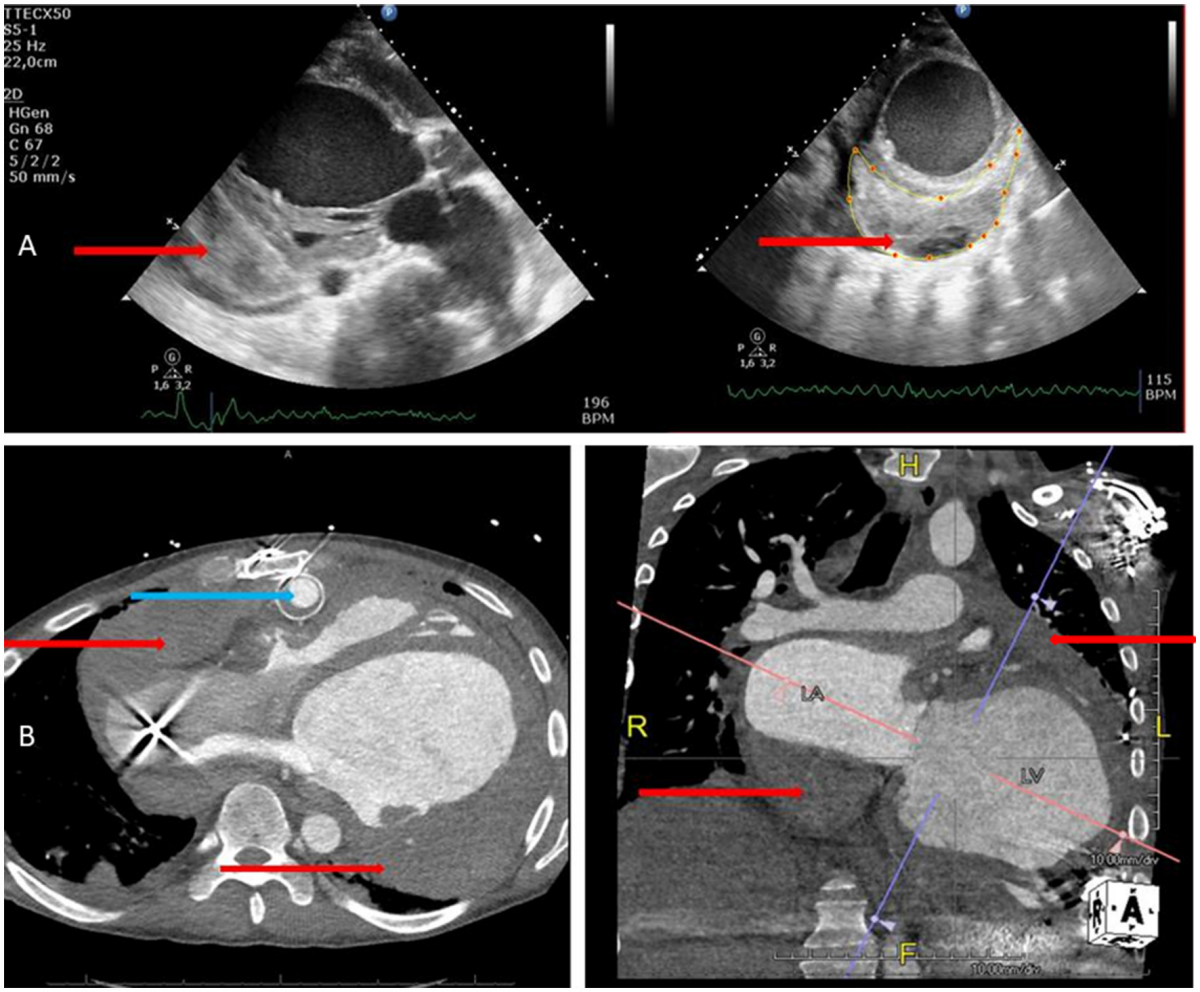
(A) Echocardiography images in parasternal long and short axis views showing thrombus formation (red arrows) in the pericardial space at the posterior area. (B) CT images of the whole thorax from transversal and anterior views showing thrombus formation (red arrows) around the left ventricle, and the atria images collected with echocardiography which are voluminous and more detailed and also show the HeartMate II outflow graft very clearly (blue arrow).
